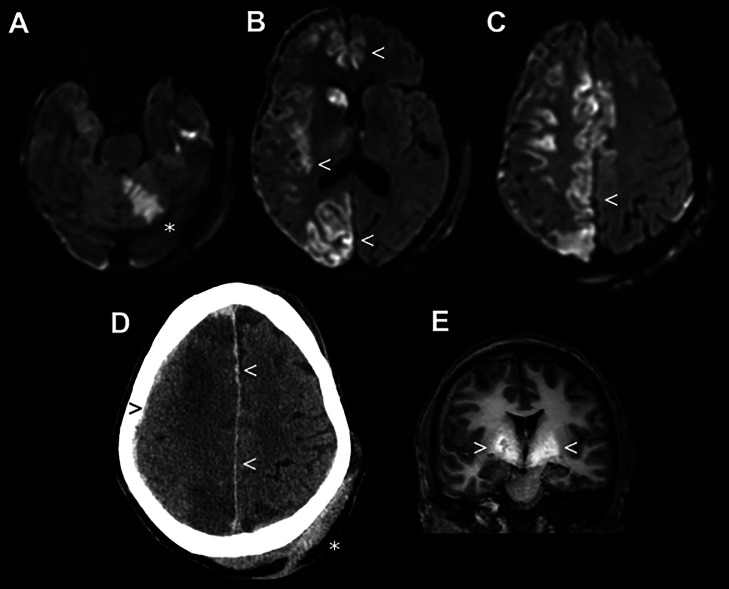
Cortical laminar necrosis (CLN) on brain MRI. (A) Acute left cerebellar stroke (*), which is the putative explanation why the patient fell and stayed down. Other brain lesions, which spared the white matter, were not congruent with arterial territories. Willis circle, brain and cervical arteries were normal (not shown). (B and C) Diffuse CLN spread over the right hemisphere and minimally diffusing over the left paramedial cortex (<). (D) Cranial computed tomography showing left subgaleal traumatic hematoma (*) and right contrecoup subdural (>) and subarachnoid hemorrhage (<). (E) Increased T1w signal in deep grey matter structures (>), typically associated with chronic hepatic encephalopathy. Axial brain MRI with DWI (A-C) and T1-weighted (E) sequences.
